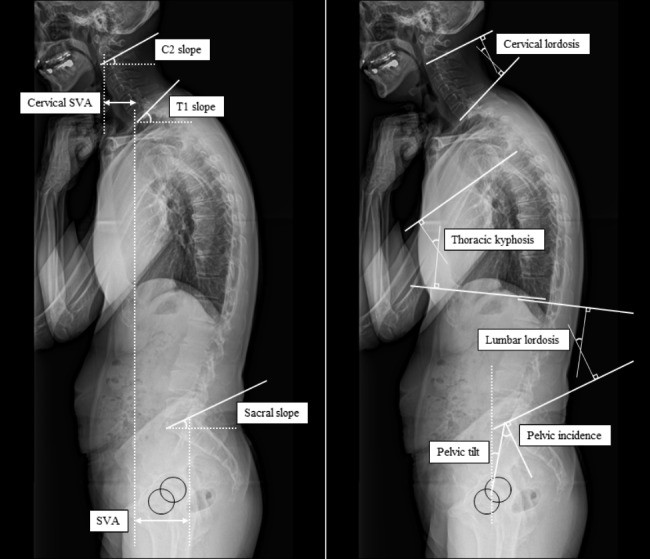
Radiographic parameters of sagittal alignment. (SVA, sagittal vertical axis)
